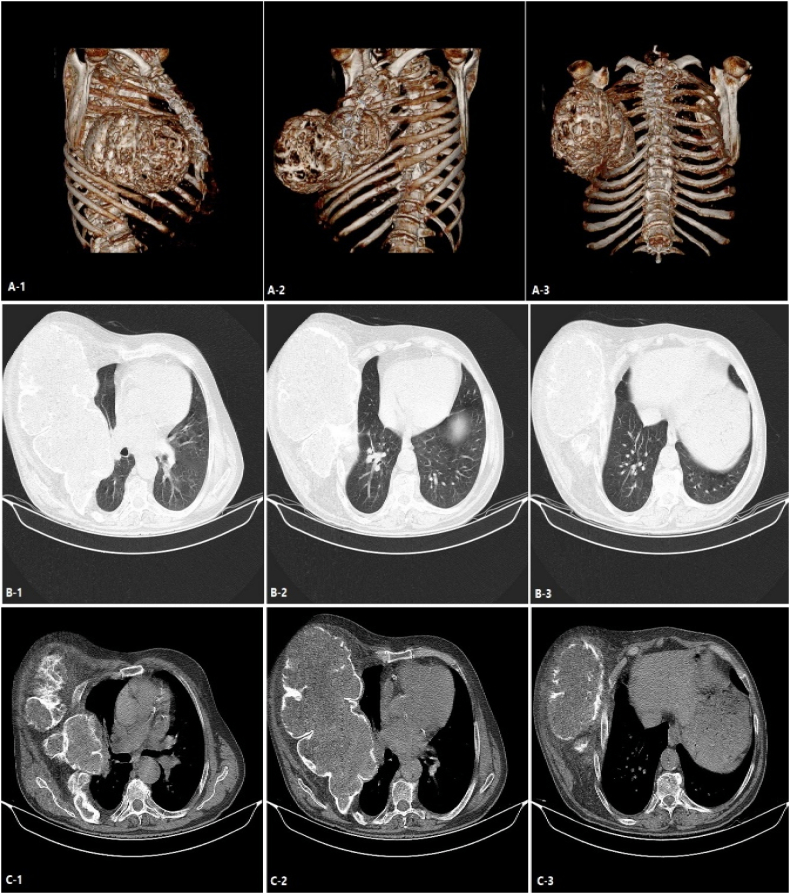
Computed tomography scan demonstrating a 36 × 18 cm mass with a rim of reactive bone. *A*1–3: Reconstructed Images. *B*1–3: Parenchymal window images *C*1–3: Mediastinal window images.
